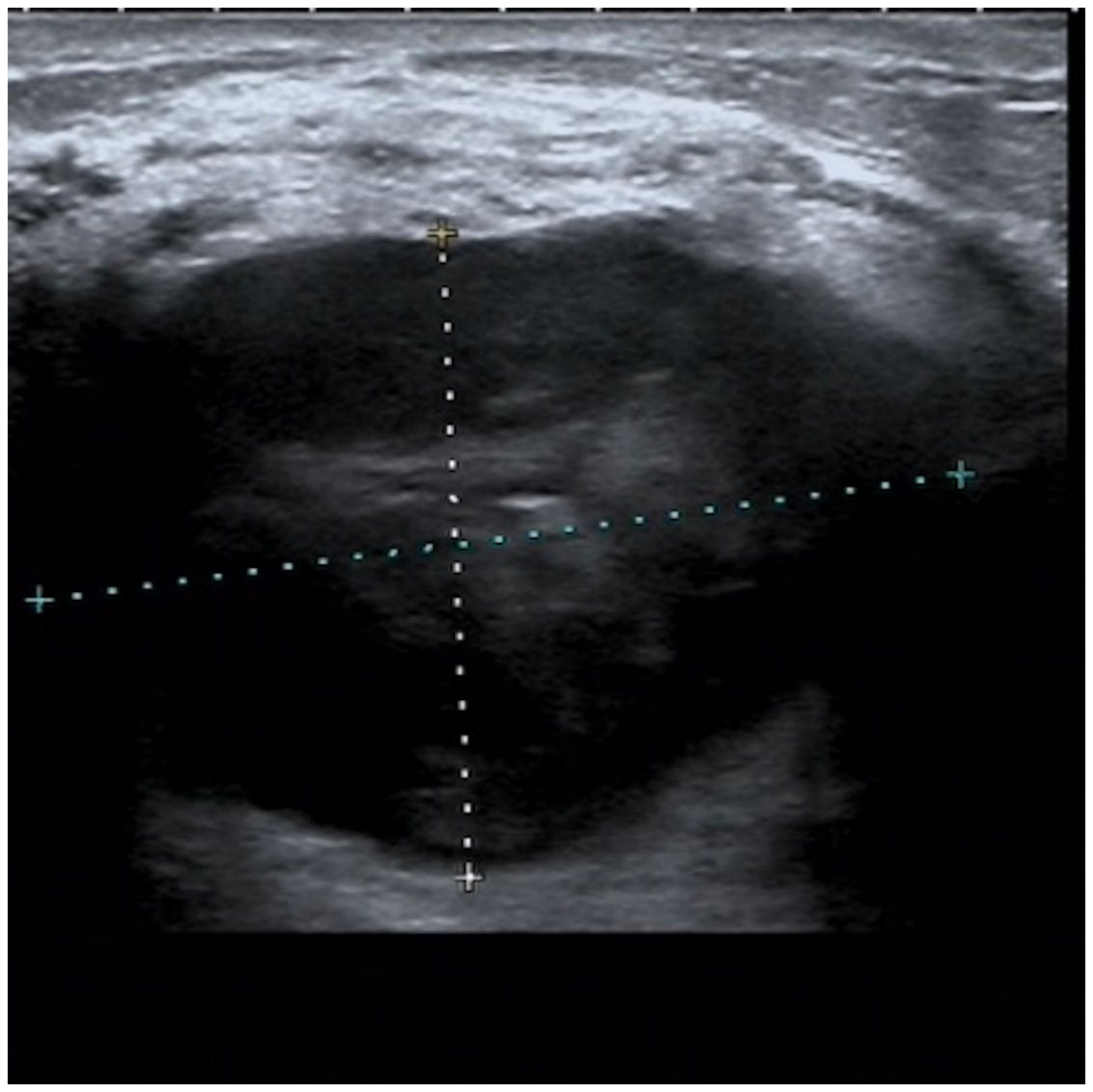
Ultrasound from June 20, 2022. A hypoechoic mass measuring 48.8*34.5 mm was observed in the upper outer quadrant of the right breast, with unclear boundaries and a relatively regular shape.
